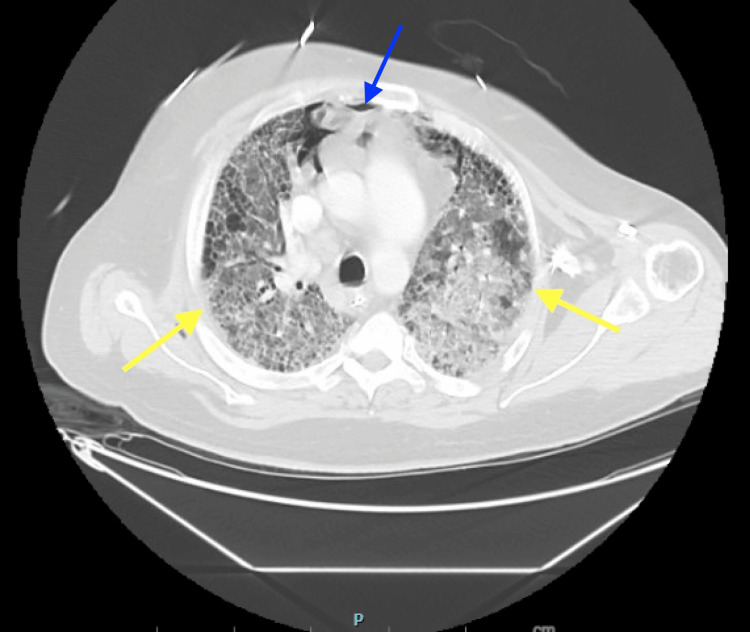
Interval CT of the chest. The image shows significant progression of interstitial lung disease with peripheral honeycombing, paraseptal emphysema, worsening bilateral ground-glass opacities (yellow arrows), and pneumomediastinum (blue arrow). CT: computed tomography.
